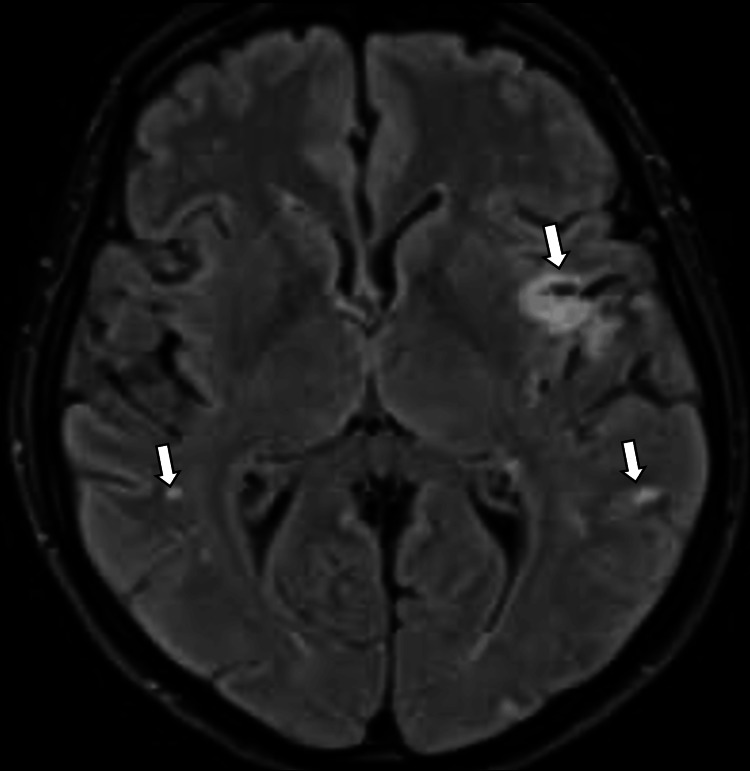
Brain MRI showing FLAIR hyperintensity and diffusion restriction within the left Sylvian cortex in keeping with acute infarct (top right arrow), small foci of infarction seen within the left occipital and parietal lobes (lower right arrow), and probable tiny infarcts within the right parietal lobe (left arrow) demonstrating multivessel territory infarcts.
